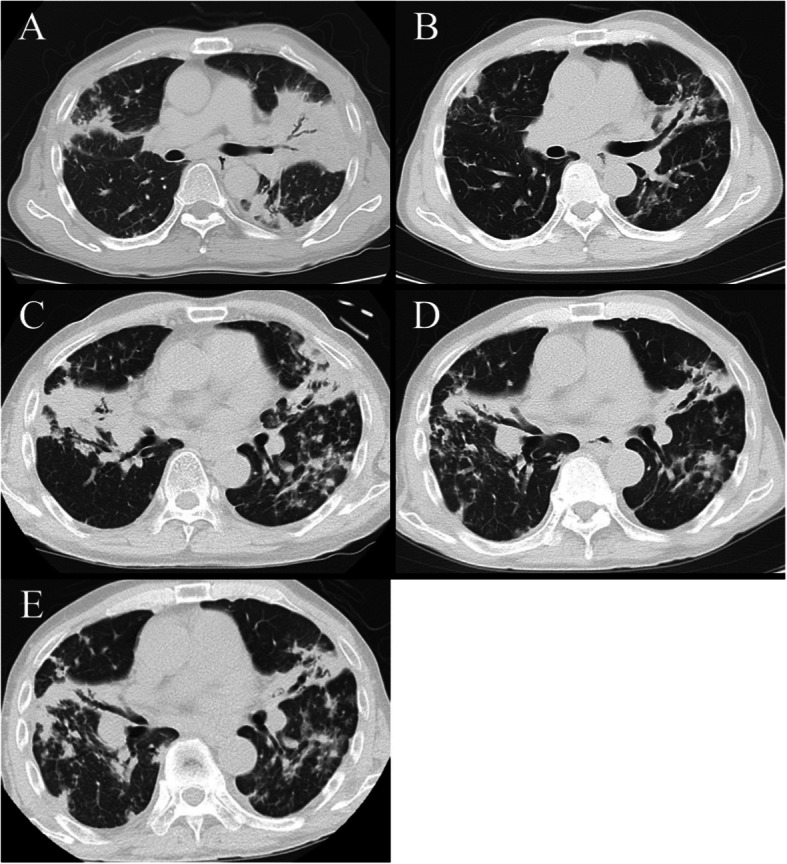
Chest computed tomography. a Computed tomography showed consolidations in the right middle and left upper lobe when *Mycolicibacterium fortuitum* was detected for the first time. b After treatment with clarithromycin and levofloxacin, consolidation improved. c Bilateral lung consolidation occurred. d After intravenous antibiotic therapy, pulmonary shadow improved. e After discharged and treated for 6 months as an outpatient
